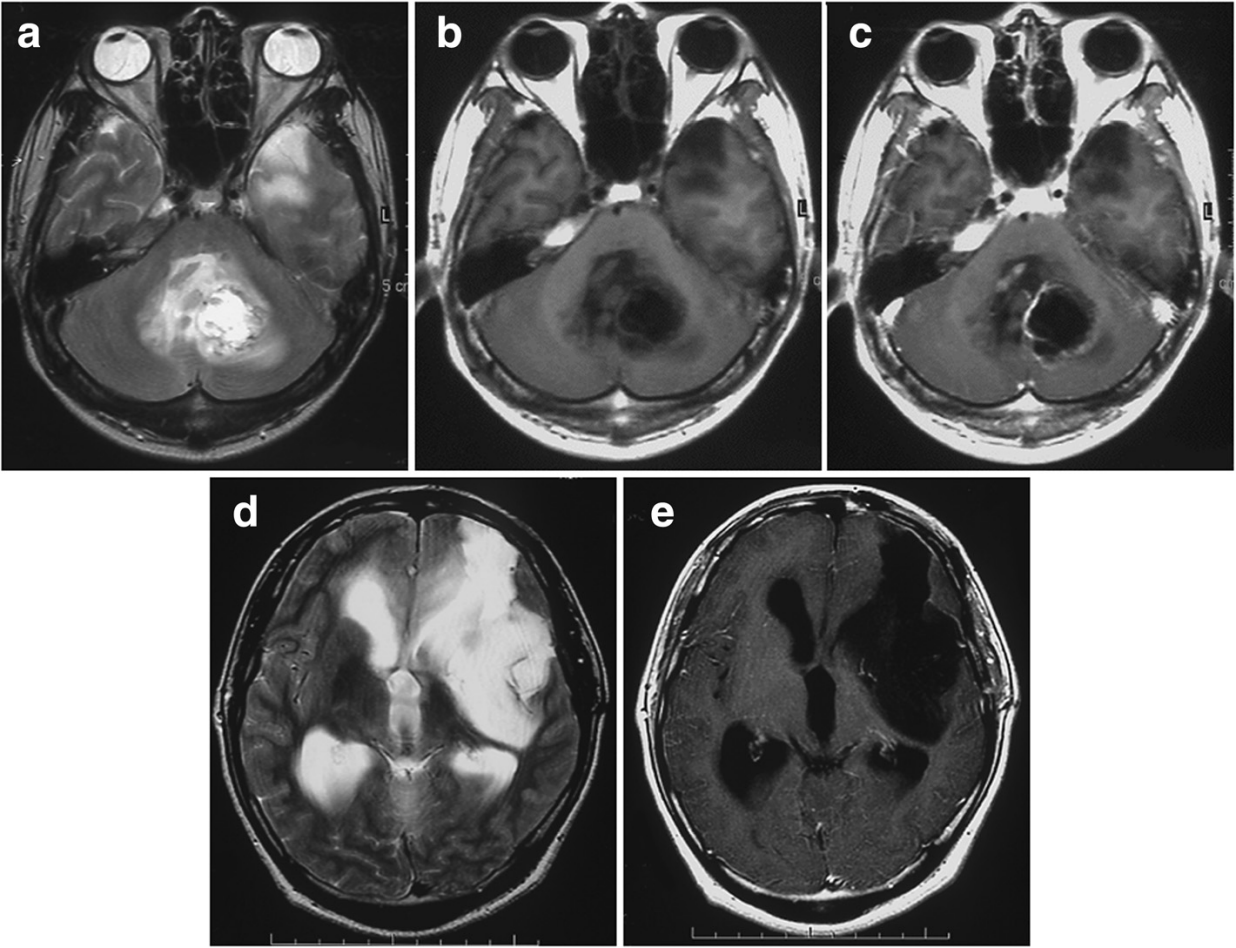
MR images at 7 months after the first surgery showing occurrence of a large tumor at the paravermian region of the left cerebellum. The tumor was enhanced in a ring-like fashion by Gd and accompanied by marked peritumoral brain edema. Axial T2-weighted (a), T1-weighted (b), and Gd-enhanced T1-weighted (c) MR images. MR images disclosing no tumor growth of the left insular tumor and hydrocephalus. Axial T2-weighted (d) and Gd-enhanced T1-weighted (e) MR images
